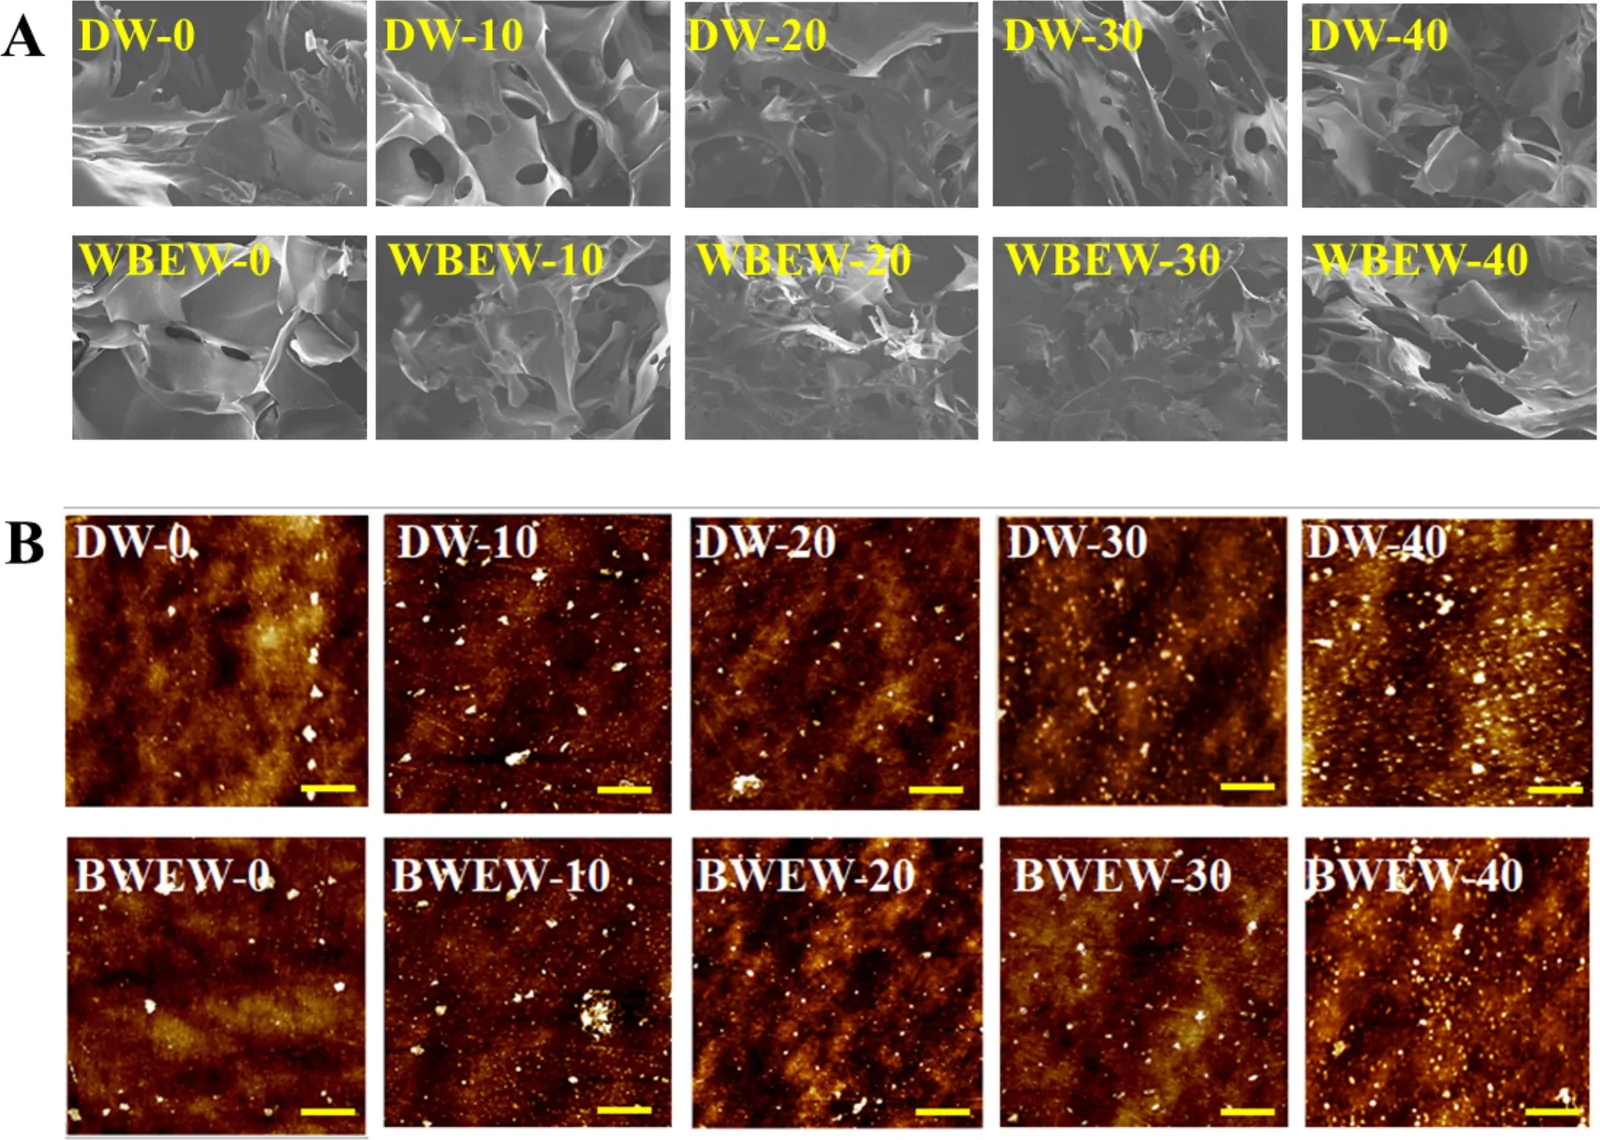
Effects of DW or WBEW coupled ultrasound treatment on the morphology (A: SEM, B: AFM) of AKP. Scale bar = 600 nm.
